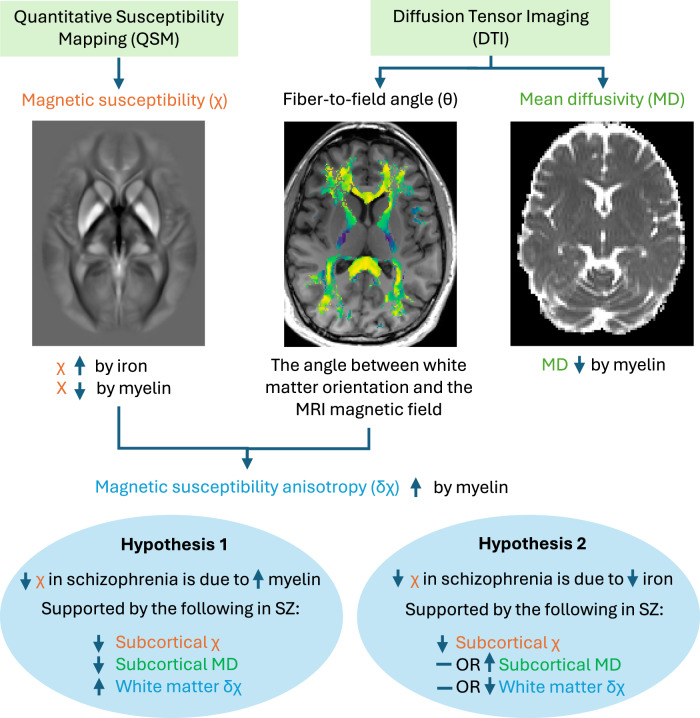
Overview of methods testing the two competing hypotheses. Quantitative Susceptibility Mapping (QSM) was used to derive magnetic susceptibility (χ), which increases with tissue iron and decreases with myelin. Diffusion Tensor Imaging (DTI) was used to calculate mean diffusivity, which inversely correlates with myelin, and the fiber-to-field angle (θ), representing the angle between predominant white matter fiber orientation and the background MRI magnetic field. Combining χ and θ enabled the calculation of magnetic susceptibility anisotropy (δχ), a marker correlated with white matter myelin. QSM and DTI were applied to healthy controls and patients to test whether low χ in schizophrenia results from high myelin (hypothesis 1) or low iron (hypothesis 2). Hypothesis 1 would be supported if patients with schizophrenia demonstrated lower subcortical χ, lower subcortical mean diffusivity, and higher white matter δχ than controls. In contrast, hypothesis 2 would be supported if patients exhibited lower subcortical χ along with either unchanged or higher subcortical mean diffusivity and either unchanged or lower white matter δχ compared to controls.
